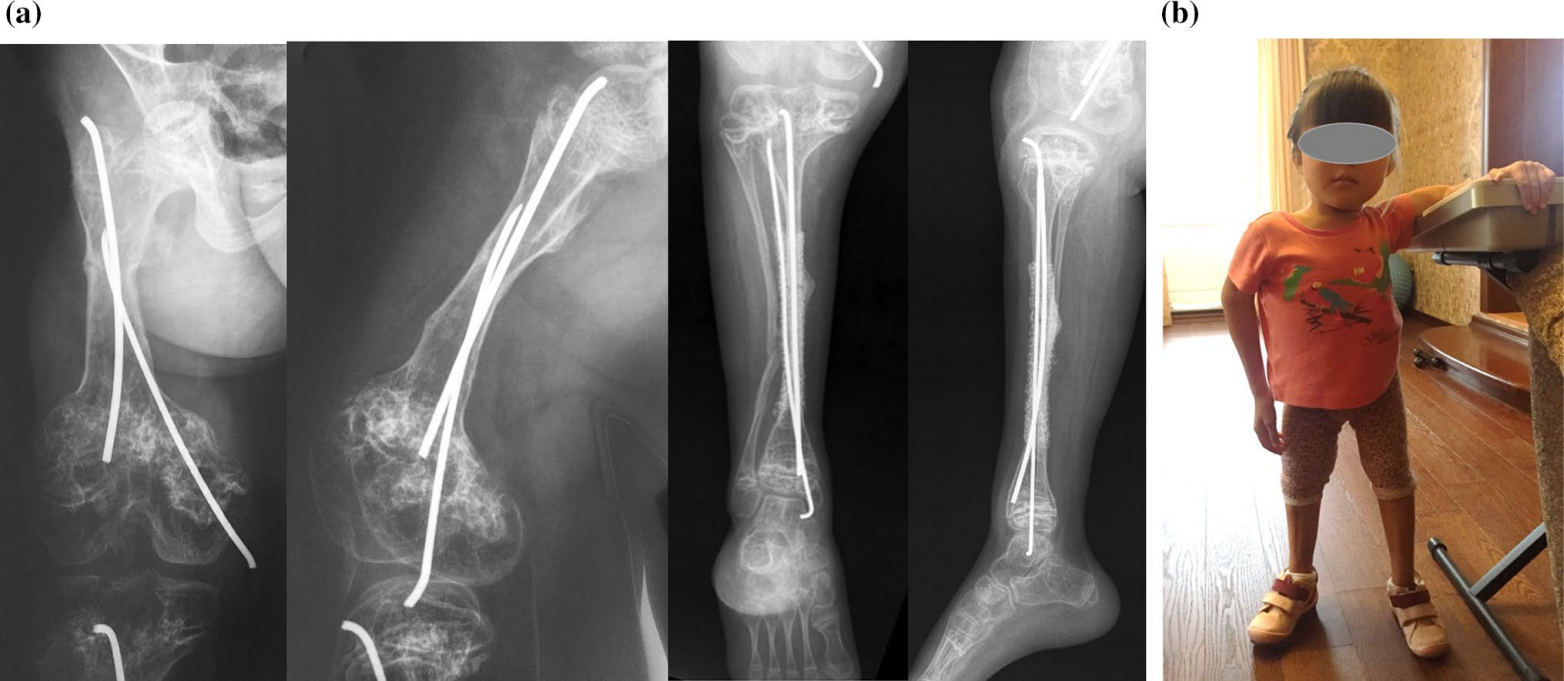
In 9 months after fixator removal: a radiographs of right femur and tibia, note remodelling of callus at femur and large periosteal callus at tibia covering titanium mesh; b patient standing with full weight-bearing
